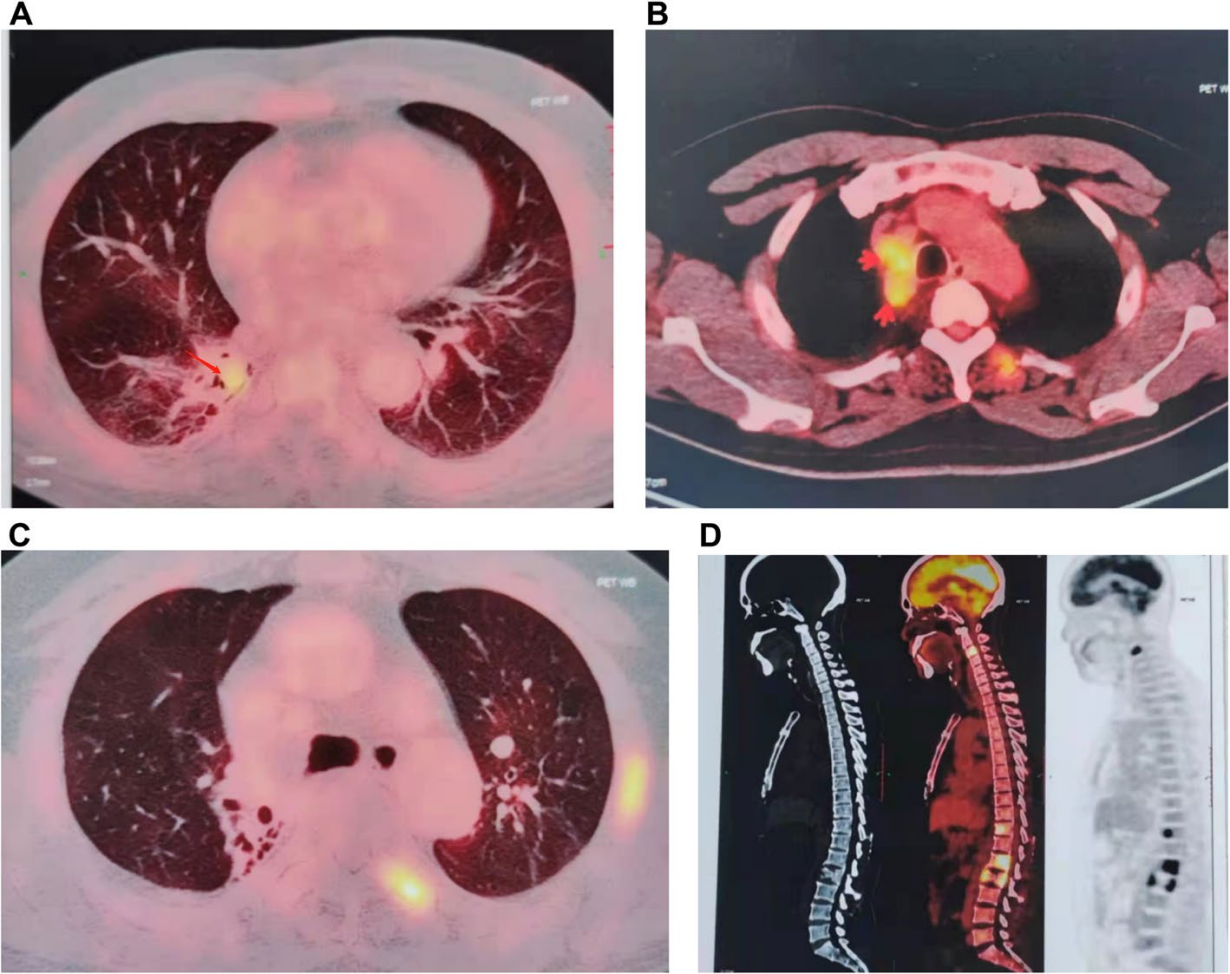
(A) PET-CT image (October 2018) displaying alveolar consolidation in the right upper lobe. B Multiple hypermetabolically enlarged lymph nodes can be seen in the right hilar and mediastinum. C–D The right clavicle, ribs, and multiple pyramids in the neck, chest, and waist showed a strong radioactive concentration shadow. CT, computed tomography; PET, positron emission tomography
